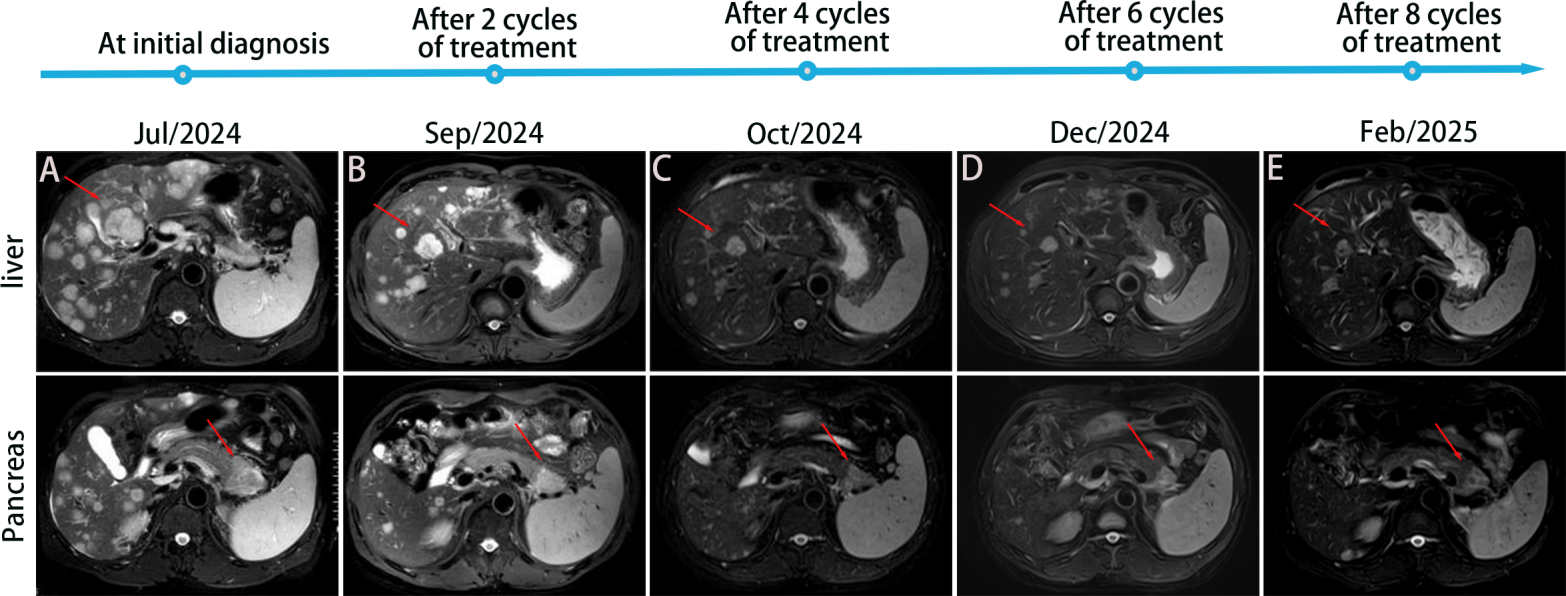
Treatment timeline and imaging follow-up. MRI T2-weighted images: (A) Diffuse intrahepatic metastatic foci before treatment (July 12, 2024), with a mass observed in the tail of the pancreas. (B) Intrahepatic metastatic foci and the pancreatic tail lesion significantly reduced and minimized after 2 cycles of treatment. (C-E) Further reduction and minimization of intrahepatic metastatic foci and pancreatic tail lesion after 4, 6, and 8 cycles of treatment, respectively.
